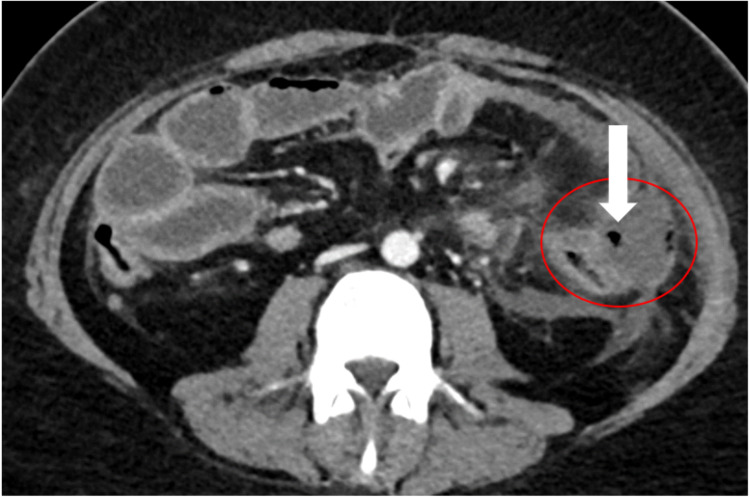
CT Image Axial image of CT scan in late arterial and early venous phase showing a 4x5 cm collection adjacent to the mid descending colon and proximal jejunal loops with multiple small air bubbles representing a bowel perforation and an infected collection (arrow). CT, computed tomography.
